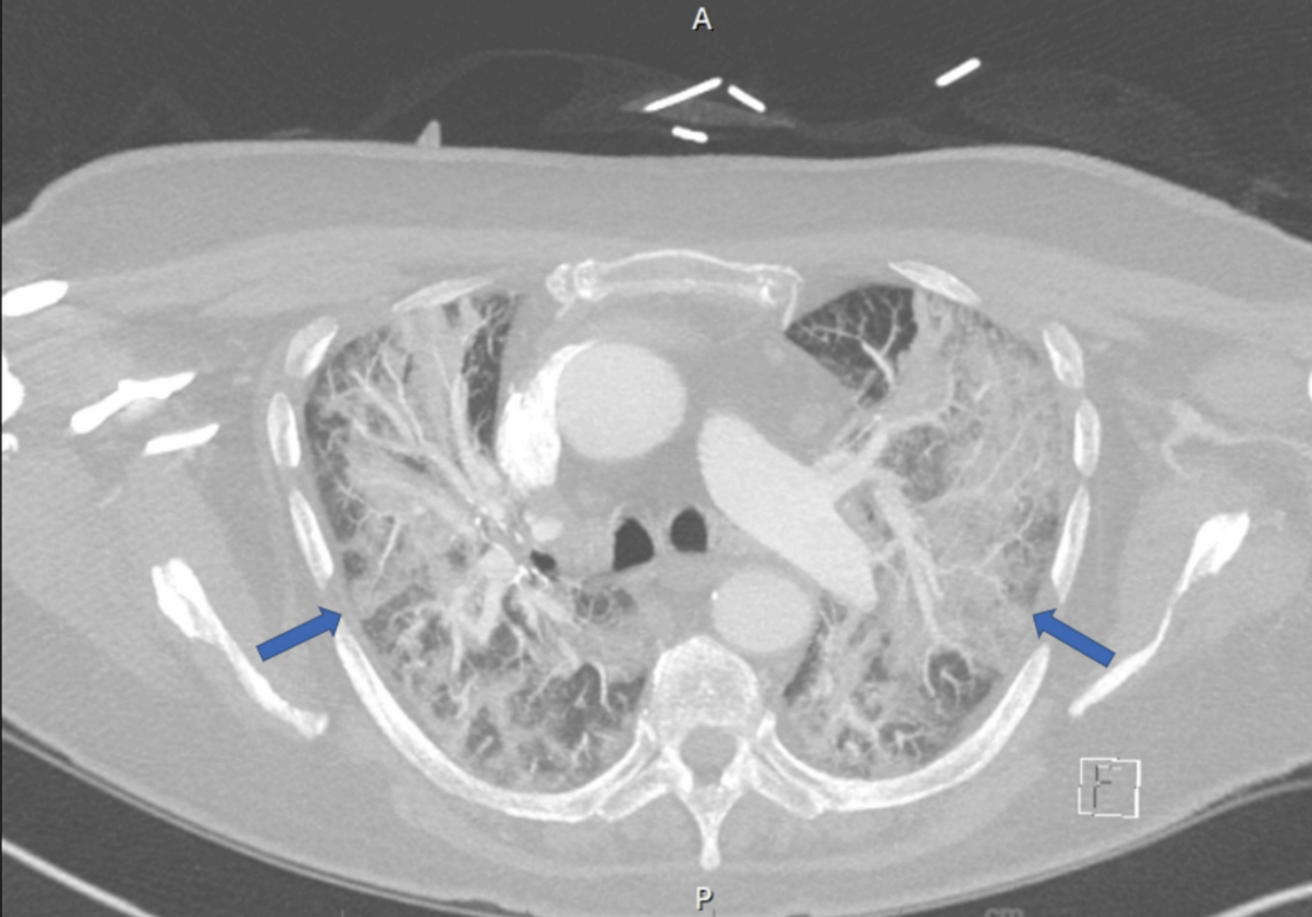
Chest CT scan shows extensive perihilar dense consolidation and ground-glass opacities with interstitial coarsening.
